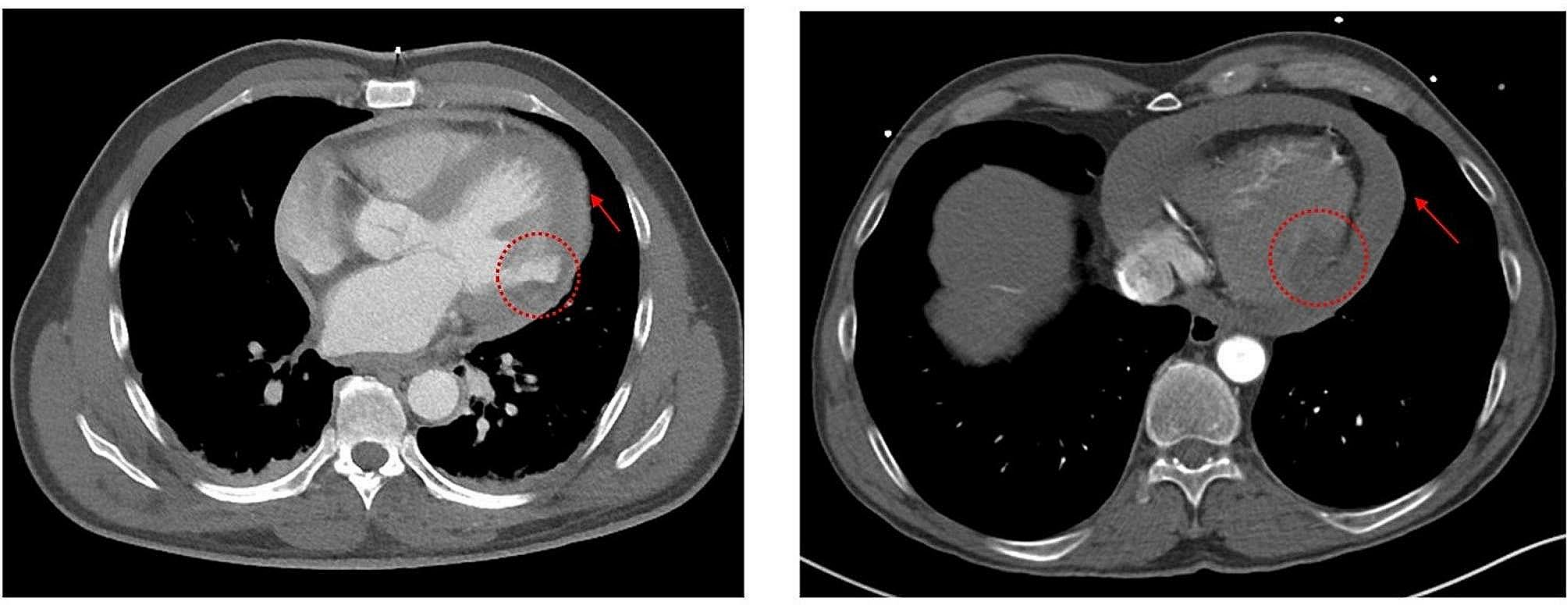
Preoperative thoracic CT-Angiogram Fig. 2A shows minimal pericardial effusion (red arrow) with suspicion of LVFWR of the first marginal branch (red circle) in patient I Fig. 2B shows relevant pericardial effusion (red arrow) and a hypocontrasted myocardial area of the first marginal branch (red circle) in patient II
